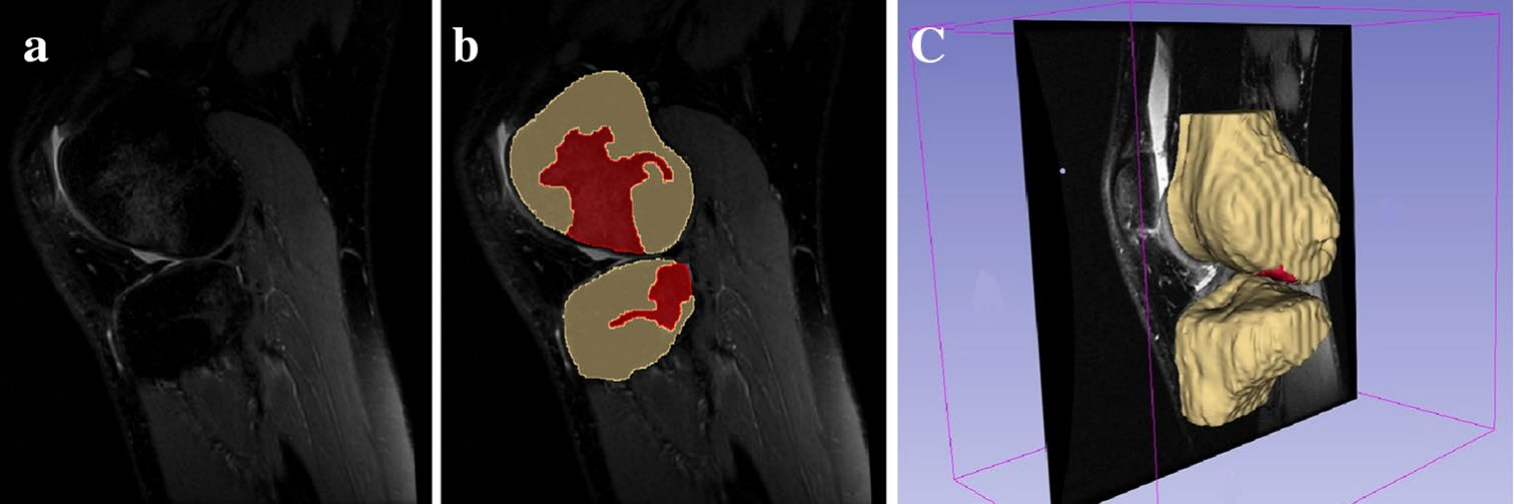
Example of segmentation process in 3D Slicer software [11]. BB were identified on MRI image of the knee (a) and underlined in every slice through segmentation tool (b), at the end of the process three-dimensional models of tibia, femur and correspondent areas with edema were obtained (c)
